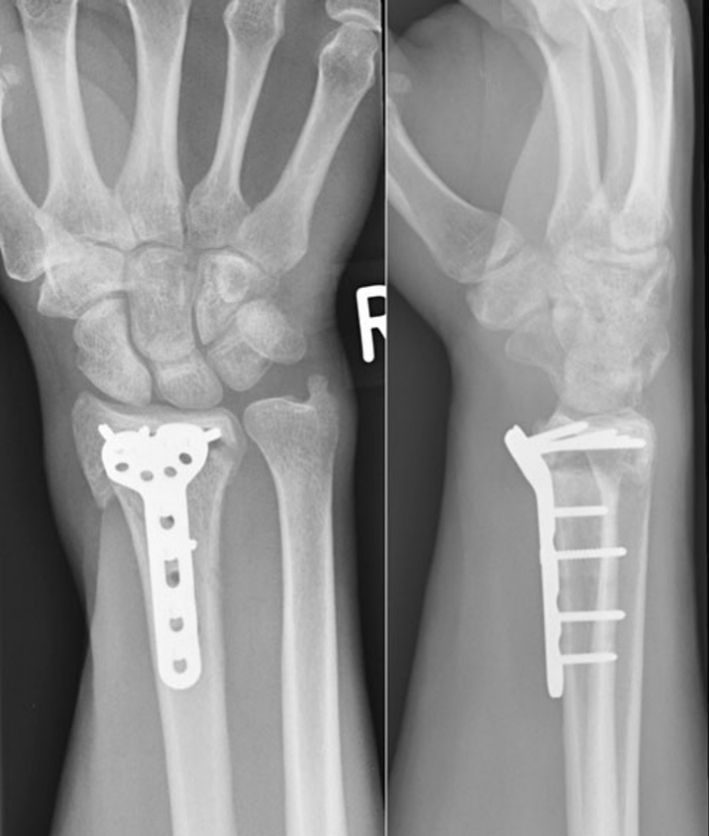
Radiographs at 20 weeks after the first revision procedure
